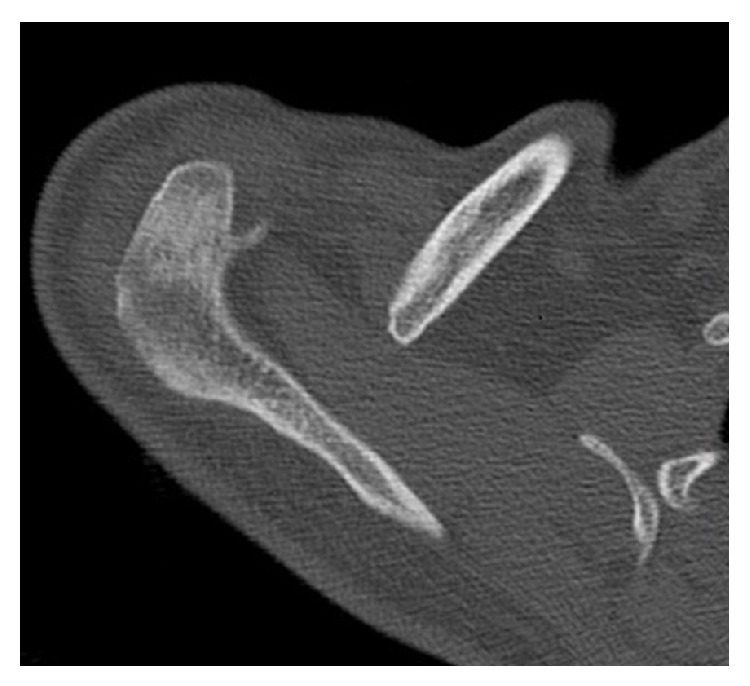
CT scan of the acromioclavicular joint demonstrating distal clavicle osteolysis compared to a normal joint.
